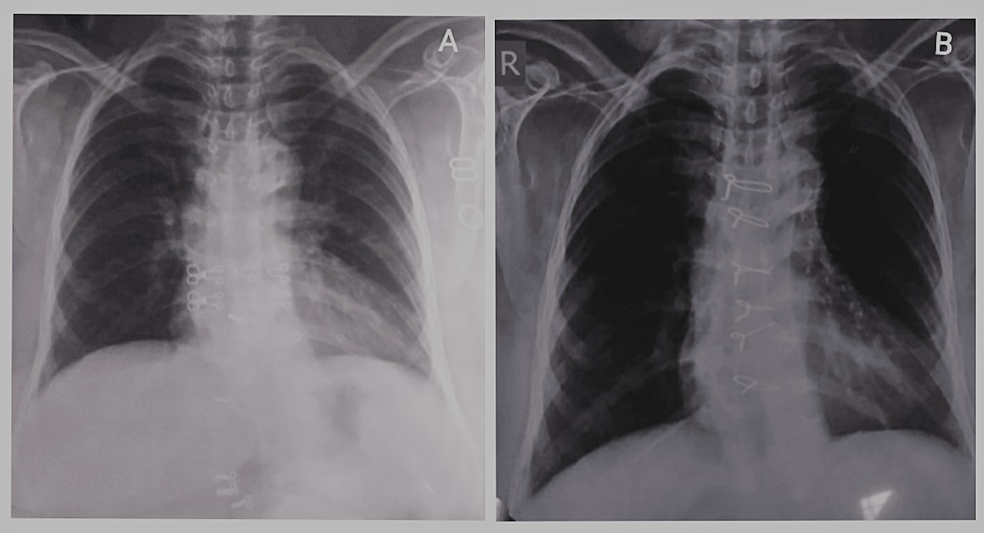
Pre-operative chest X-ray showed cardiomegaly (A) and in post-operative chest X-ray, sternal wires and cardiomegaly were noted (B).
